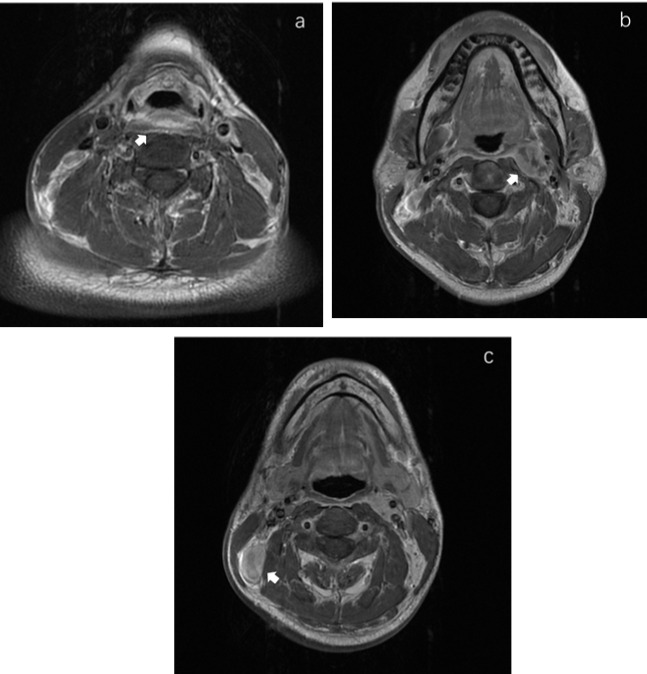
MRI images in T1 with contrast of a posterior wall cancer patient. (A) The primary focus of posterior wall cancer. (B) The left retropharyngeal lymphnode metastasis. (C) The metastatic lymphnode in right Level II.
